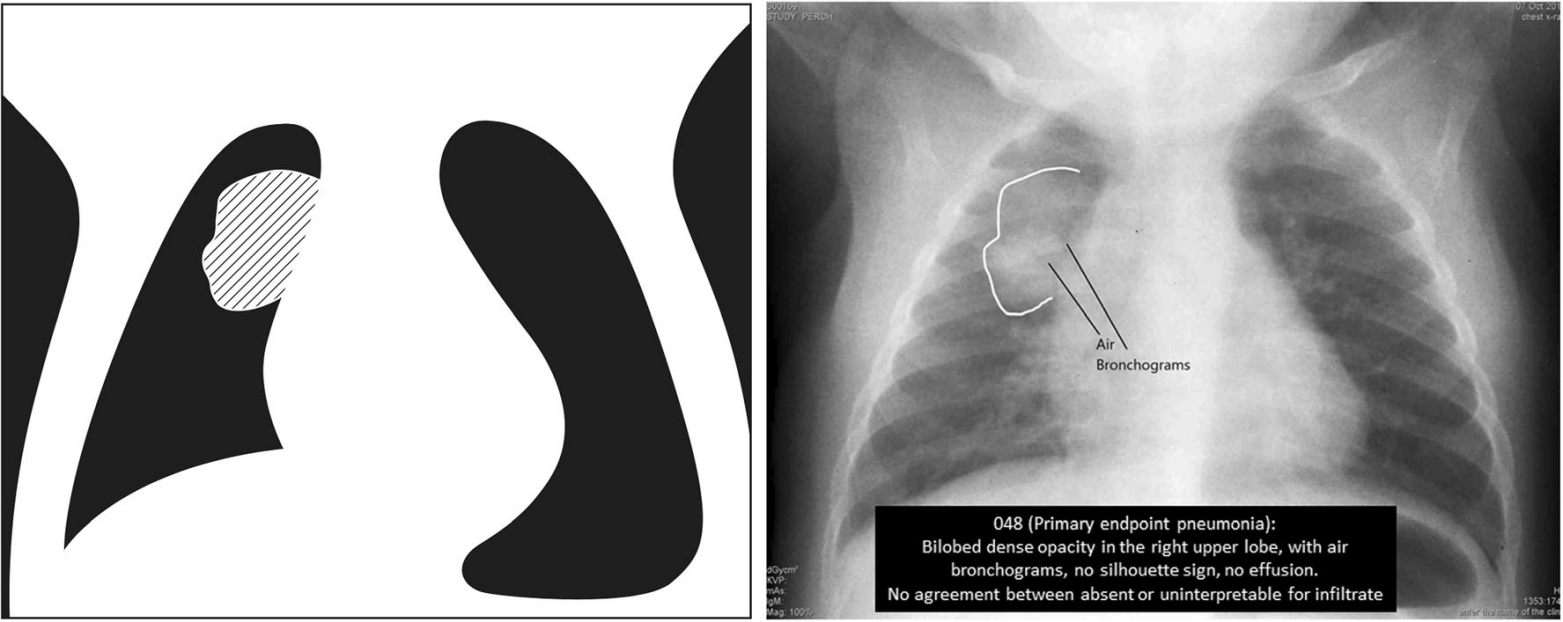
World Health Organization (WHO) Chest Radiography in Epidemiological Studies example of teaching materials for a chest radiograph with features of WHO-defined primary endpoint pneumonia
